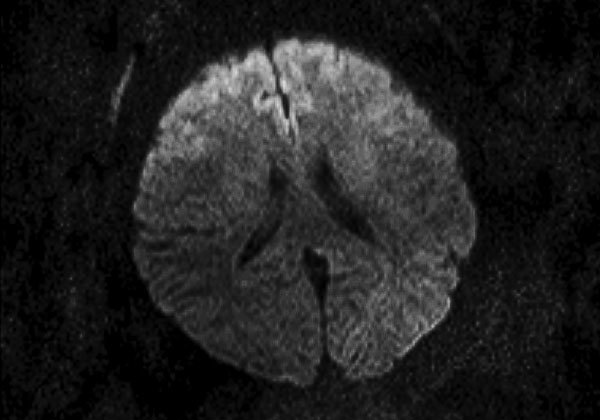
Magnetic resonance imaging of the brain of a 55-year-old woman (patient 3) who had neurologic complications of influenza B virus infection, Romania. Axial diffusion-weighted image showing restricted diffusion in the bilateral frontal cortex.
